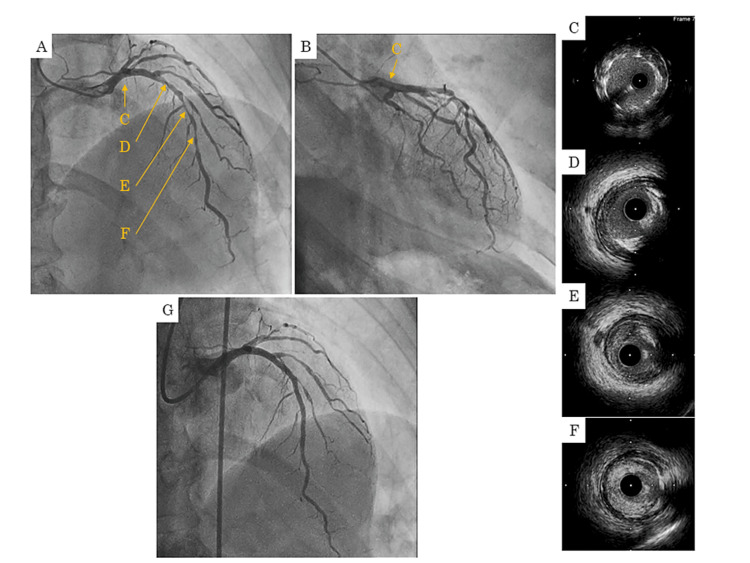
(A, B, C) On day 17, the thrombus in the LMT disappeared according to angiography and IVUS findings. (D, E, F) According to IVUS findings, the lesion in #6-7 primarily consisted of a mixture of fibro-fatty and fibrous plaque with some calcified plaque. (G) Deployment of drug-eluting stent into the lesion resulted in favorable dilation without evident complications. (C, D, E, F) IVUS findings LMT: left main trunk, IVUS: intravascular ultrasound
